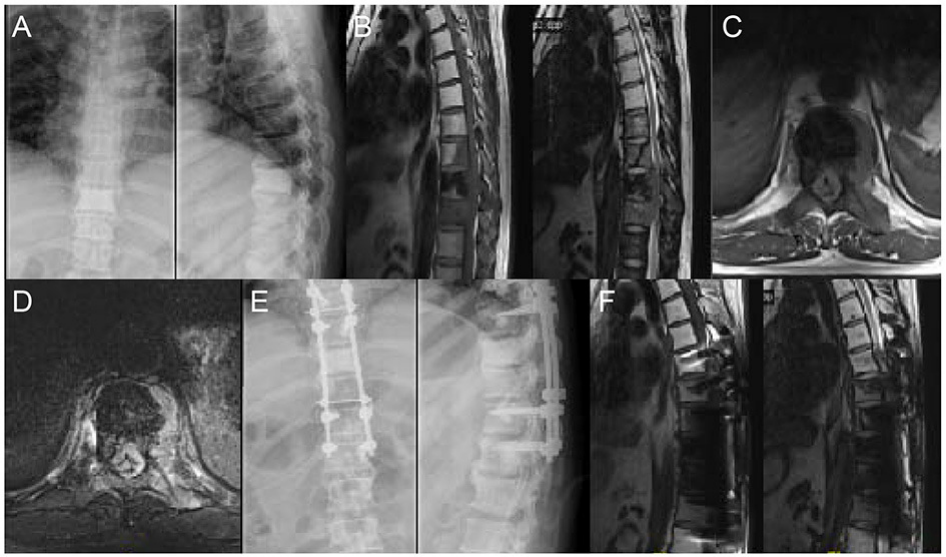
A 48 years old man who underwent surgery of lung cancer 6 years ago complained of increasing osphyalgia during the previous 1 month and was found to have metastatic lesion in T8-10; His preoperative Frankel grade was D. (A) Preoperative anteroposterior and lateral radiograph revealed abnormal bone mineral density in T9. (B–D) Sagittal and axial magnetic resonance imaging (MRI) showing signal intensity abnormalities in T8-10 and a pathological fracture of T9. (E) Postoperative anteroposterior and lateral radiograph. (F) Postoperative sagittal MRI.
